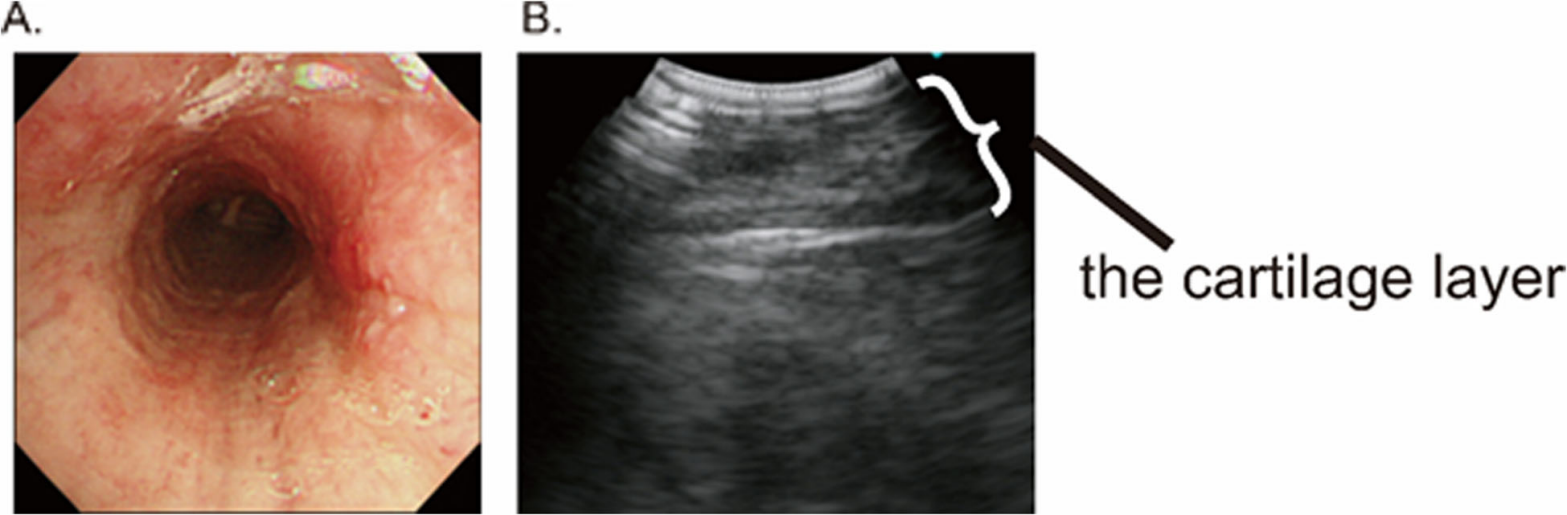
Imaging findings of bronchoscopy. a Bronchoscopy shows narrowing of the trachea and the straight pattern on the membranous portion with disappearance of the tracheal cartilage. b Endobronchial ultrasonography (EBUS) of trachea shows thickening of the cartilage layer
